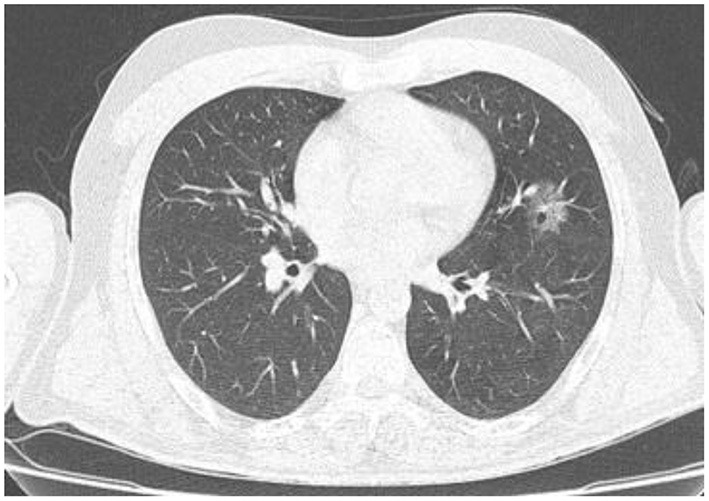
High resolution, axial non-enhanced spiral chest CT image (lung window) of a 44-years old patient who was confirmed to be infected by COVID-19 and admitted to hospital with no symptoms (asymptomatic). CT image shows only a small ground-glass pattern in the lower aspect of the anterior segment of the upper lobe of the left lung.
